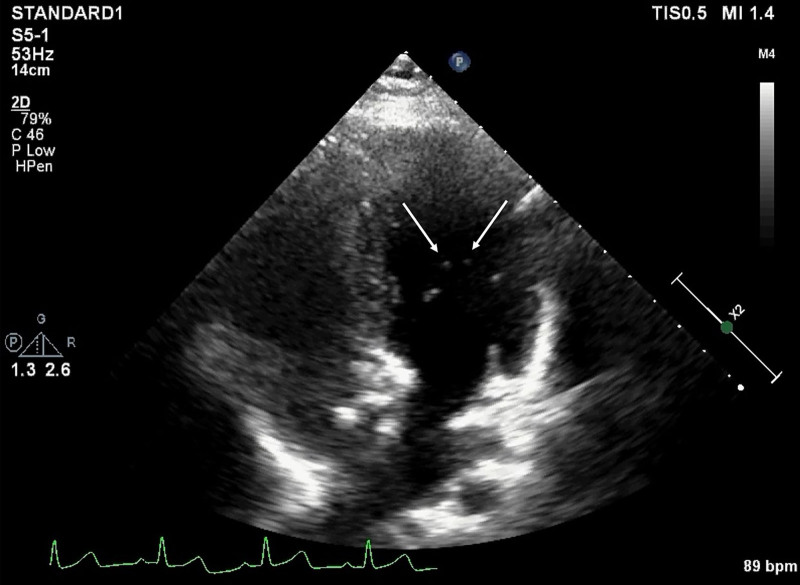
Less than 5 microbubbles were found at the left-sided heart chambers (arrows) after effusion of the right-sided heart chambers showed by agitated saline contrast transthoracic echocardiography through the left median cubital vein.
